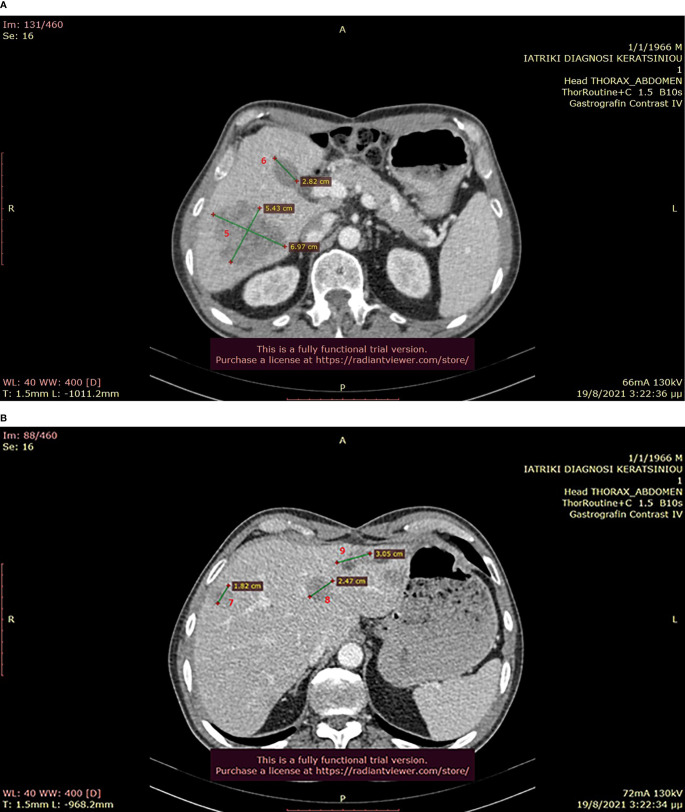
(A, B): Abdominal CT after the completion of 6 treatment courses, showing SD in the liver (August 2021).
